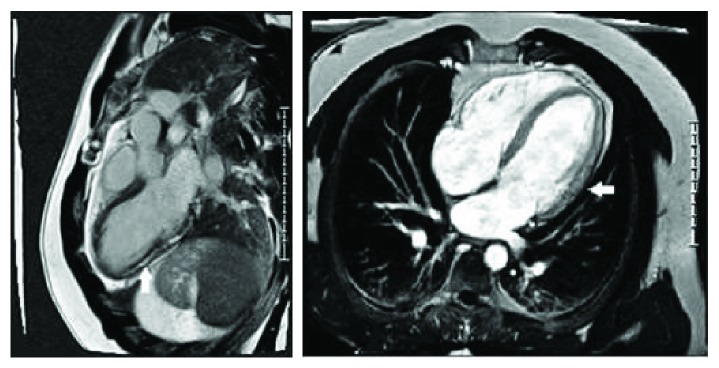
Cardiac MRI showing extensive subepicardial and midwall late enhancement typical of myocarditis in the anterior, lateral, and inferior walls.
